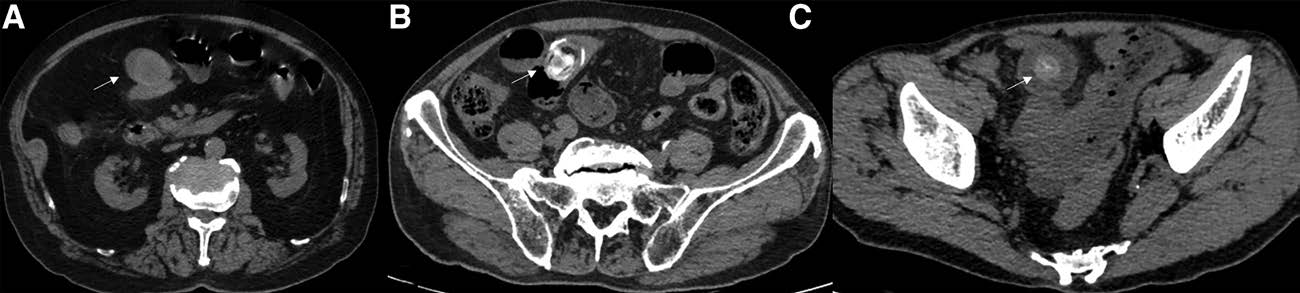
CT scans in the axial plane show three gallstones in the small bowel segment (arrow). (A) A round, high-density stone of a 91-year-old-man in ileum. (B) An oval stone of a 74-year-old-man at the junction of jejunum and ileum appears to be high-density mixed with low-density. (C) A round, high-density stone of a 71-year-old-man in ileum. CT = computed tomography.
